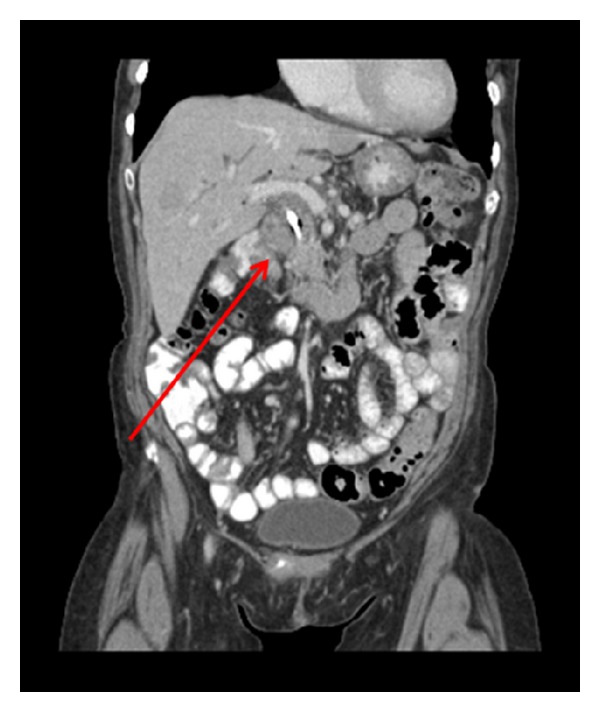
Contrast-enhanced coronal CT image demonstrates an ill-defined mass in the porta hepatis causing compression of the portal vein and common bile duct (red arrow).
